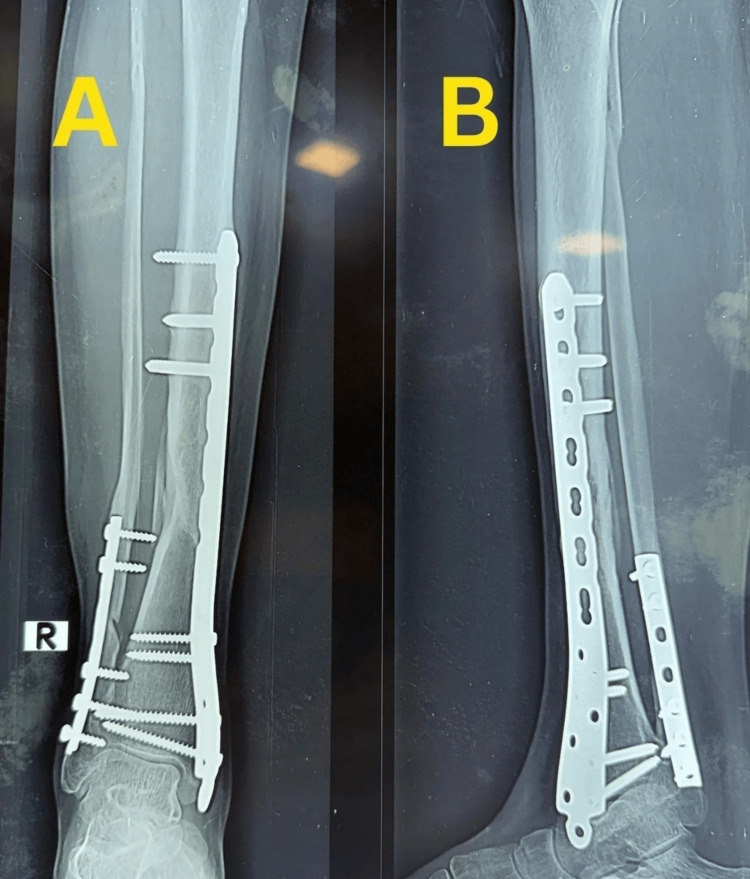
Follow-up radiographs showing fracture healing in Case 3. (A) Anteroposterior view demonstrating maintained alignment with bridging callus formation at the fracture site. (B) Lateral view showing progressive healing without implant loosening or loss of reduction.
